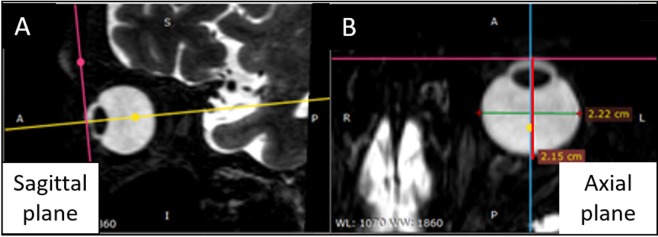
Definition of axial length and transverse length on MRI. (A) On the sagittal multi-planar reconstructed plane, the axial plane was defined as the horizontal plane connecting between the top of the cornea and the optic disk. (B) On the axial/horizontal plane, the axial length (red line) was defined as the distance between the top of the cornea and the rear part of retina (approximately in the foveal region) in the vertical direction tangential to the corneal curve. The maximum distance orthogonal to the eye axis was defined as the transverse length (green line).
